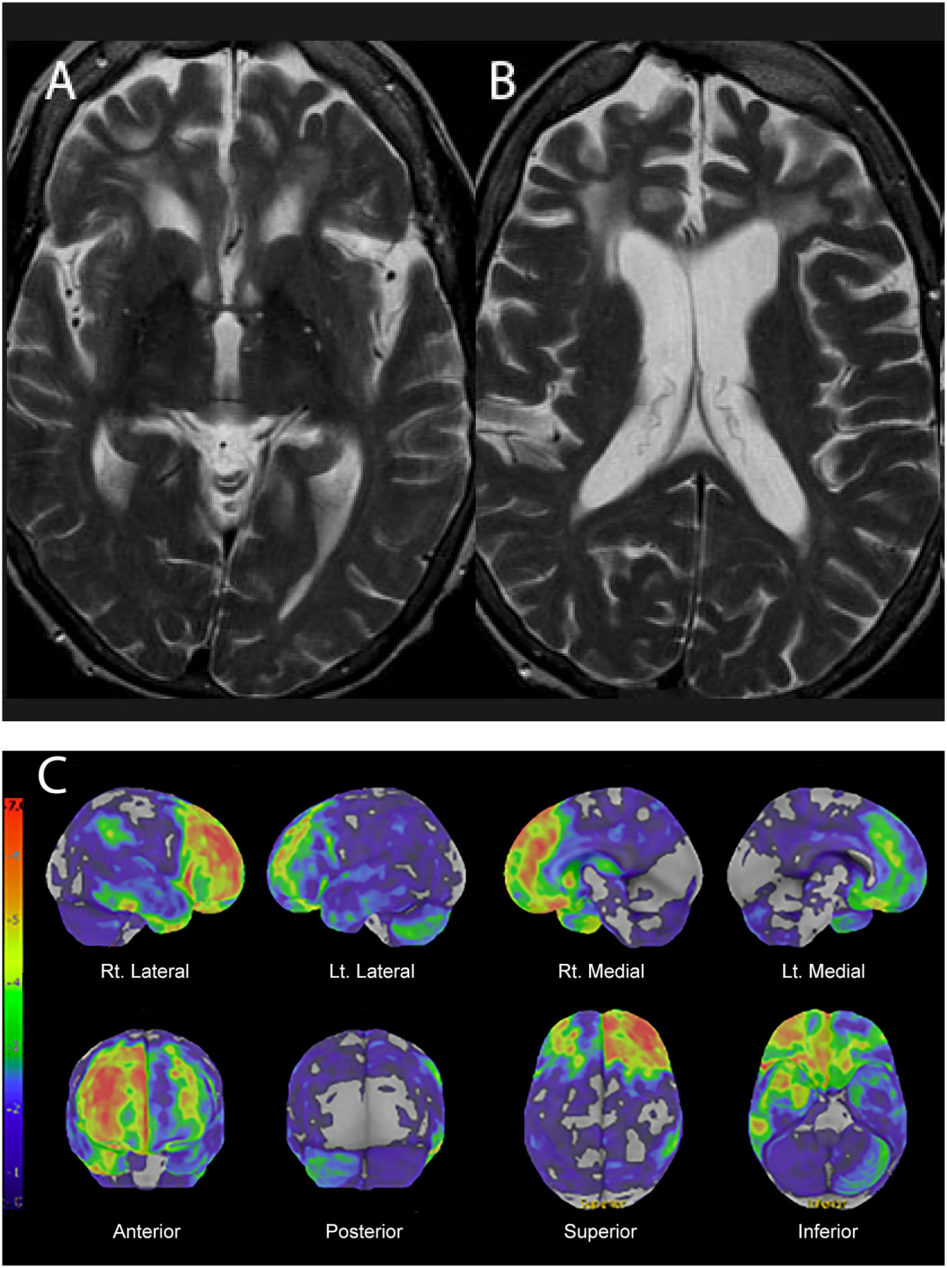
63-year-old woman with cognitive decline and unusual aggressive behavior. T2-weighted axial image at the basal ganglia (A) and the lateral ventricle (B) shows frontal dominant cortical atrophy with a moderate amount of white matter ischemic changes. FDG-PET with 3D-SSP reconstruction (C) shows profound frontal hypometabolism, right greater than left, with preservation of the posterior cingulate and parietal lobe. The findings are consistent with behavioral variant FTD
